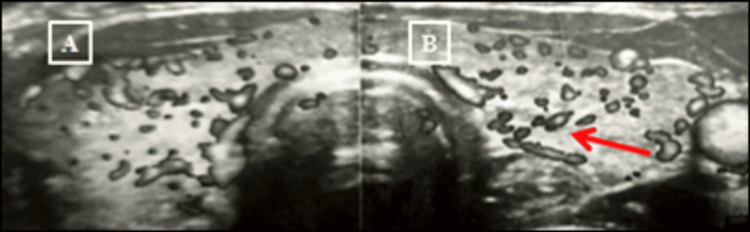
Thyroid ultrasound with features of autoimmune thyroiditis (A) Transverse ultrasound image of the right thyroid lobe; (B) Transverse ultrasound image of the left thyroid lobe. Both lobes exhibit a hypoechoic, inhomogeneous echotexture with increased vascularity, consistent with autoimmune thyroiditis. The red arrow highlights the vascular pattern.
